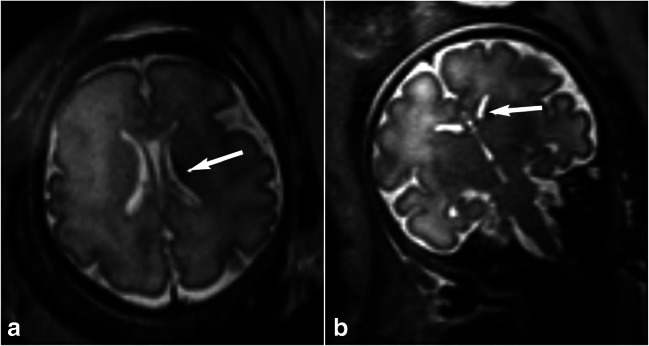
Grade 1 germinal matrix haemorrhage in a 33^+1^ weeks gestational age fetus. a, b Axial (a) and coronal (b) dynamic T2 MR images acquired in a healthy volunteer for a research study show a small unilateral focus of T2 hypointensity in the caudothalamic groove suspicious for a grade 1 germinal matrix haemorrhage (*arrows*)
